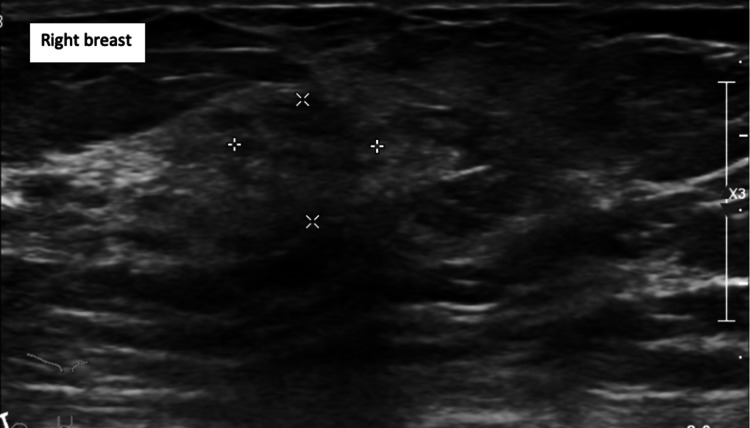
Ultrasonography of the right breast showing U4 lesion
